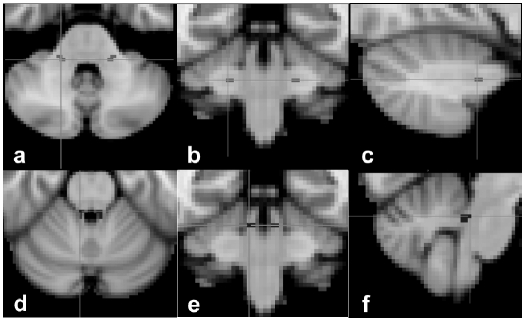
The seed masks of the middle cerebellar peduncle (a)–(c) and superior cerebellar peduncle (d)–(f) overlaid onto the MNI-152 T1-weighted brain image, in axial view (a) and (d), coronal view (b) and (e), and sagittal view (c) and (f).
